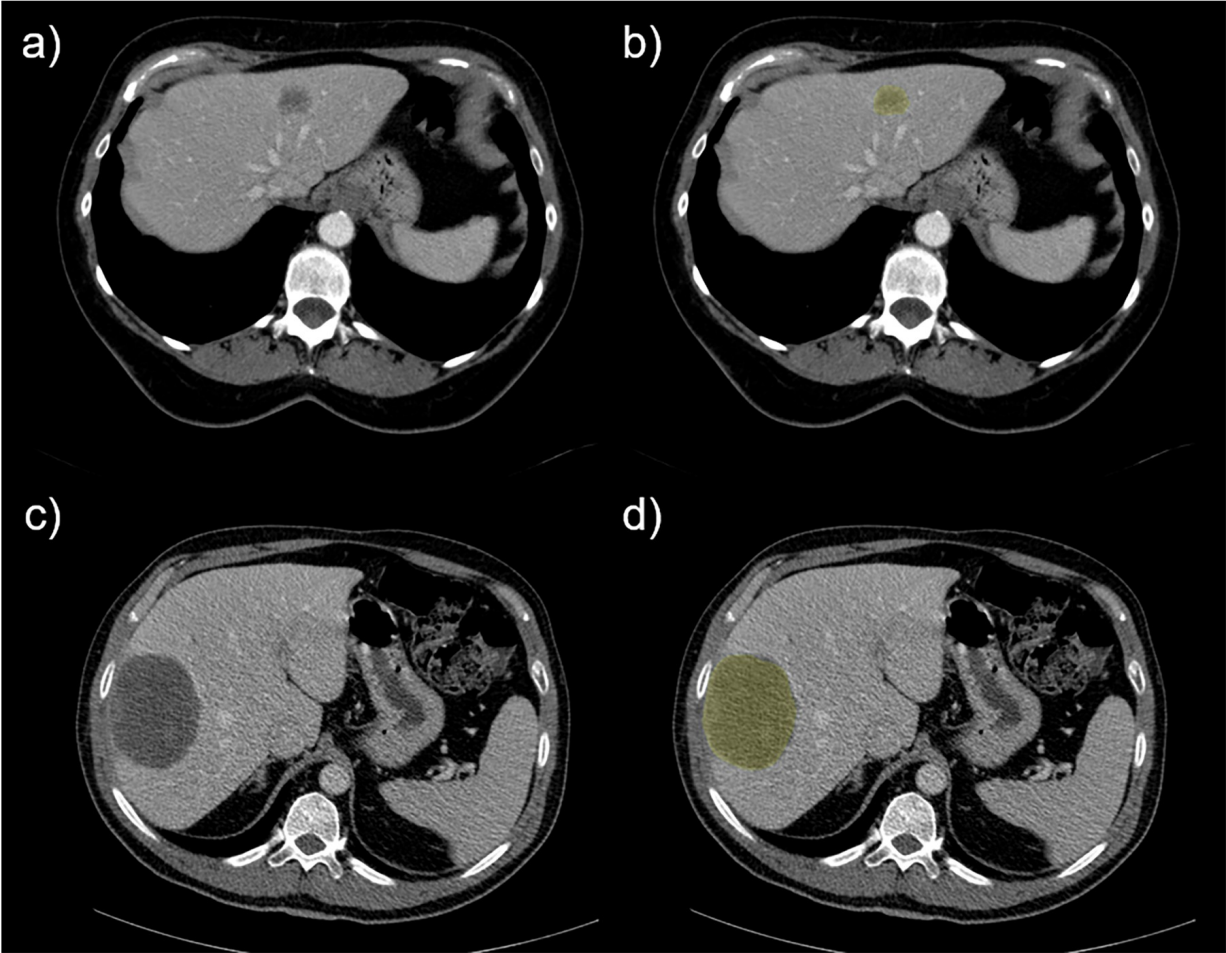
Liver metastases examples with their associated segmentations. First row exhibits (A) initial CT image (left) and (B) a segmented metastasis (yellow area) of the patient with the shortest survival in our dataset. Second row exhibits (C) initial CT image (left) and (D) a segmented metastasis (yellow) of the patient with the longest survival in our dataset.
